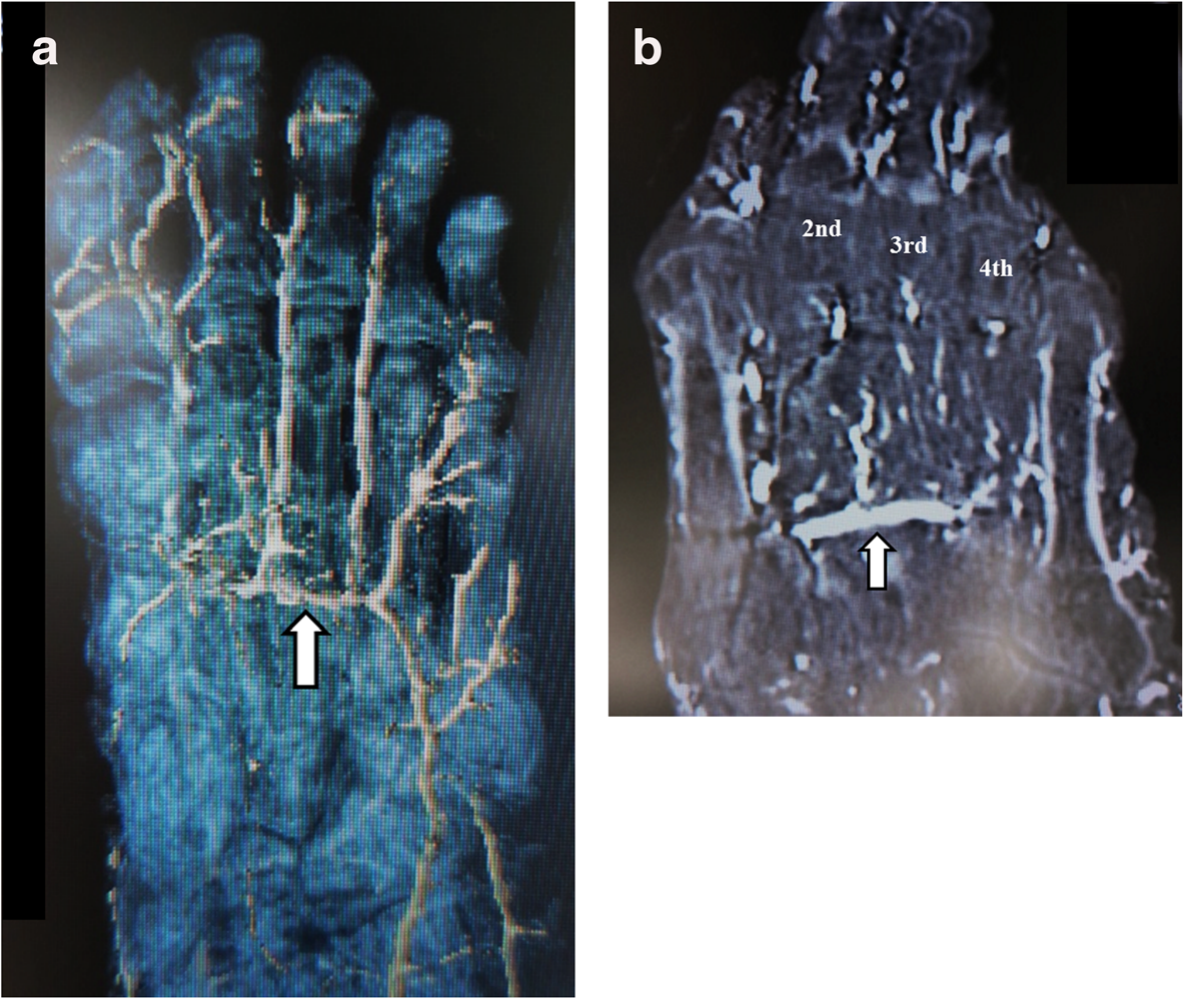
CT images showing continuity of the deep plantar arch under the lesser metatarsals. a A three-dimensional enhanced CT image viewed from the plantar aspect. b An enhanced CT image in the coronal plane (b). The deep plantar arch is indicated by the arrow. CT, computed tomography
